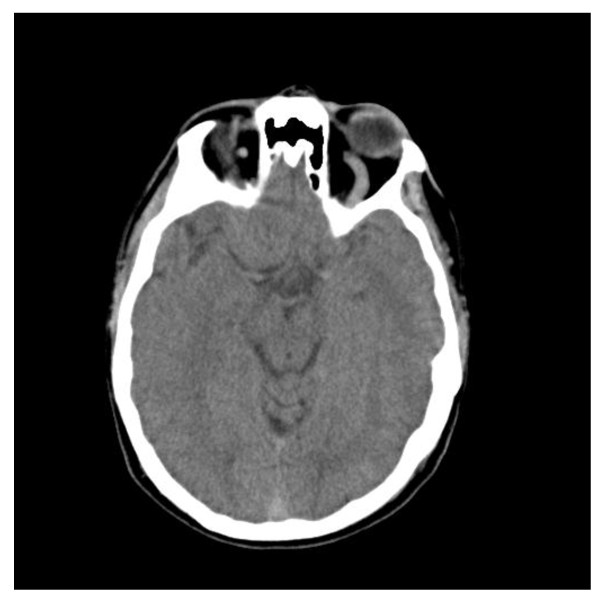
CT of the head without contrast. Left ophthalmic vein is dilated.
